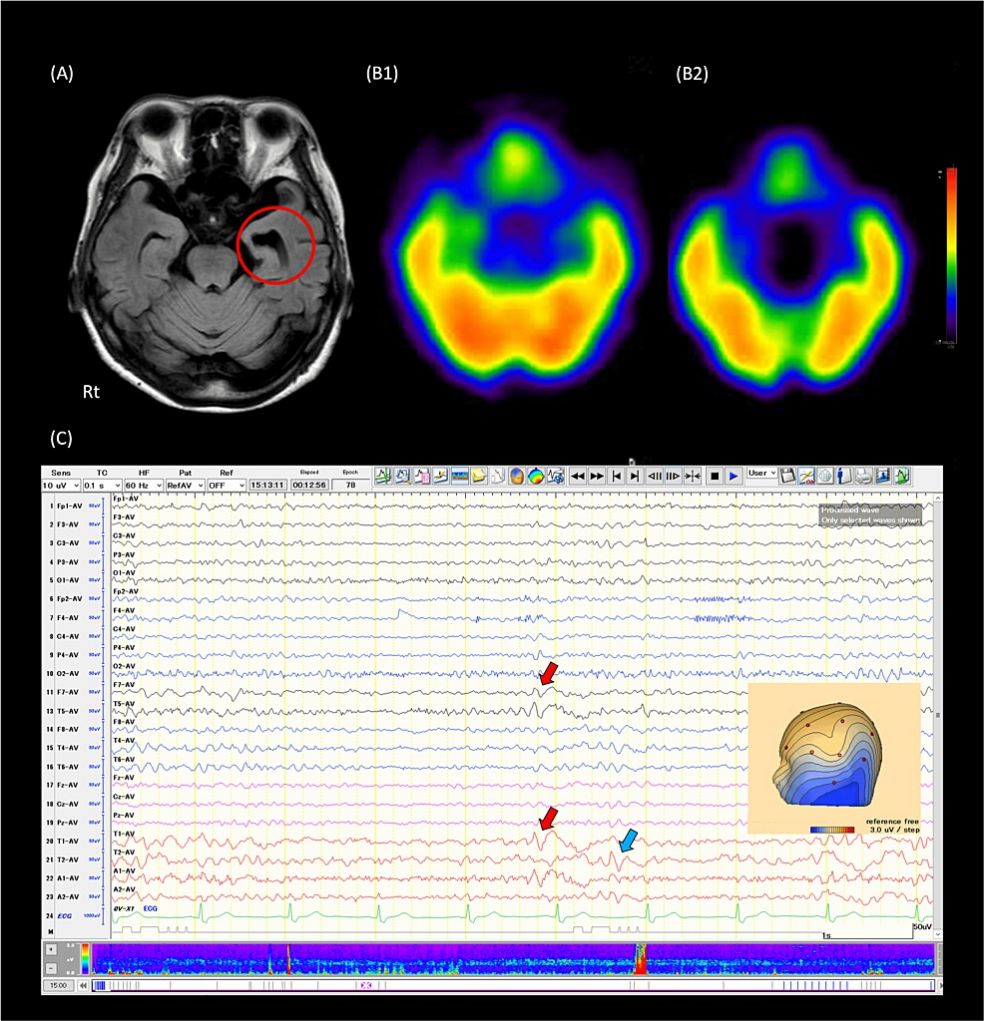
Brain imaging and interictal EEG A. Brain MRI with FLAIR imaging demonstrates atrophy of the medial temporal lobe on the left; B. 123I-iomazenil SPECT (B1: early and B2: late phase) reveals findings compatible with left temporal lobe epilepsy; C. Interictal EEG displays bilateral independent focal sharp waves, predominantly on the left. FLAIR: fluid-attenuated inversion recovery
